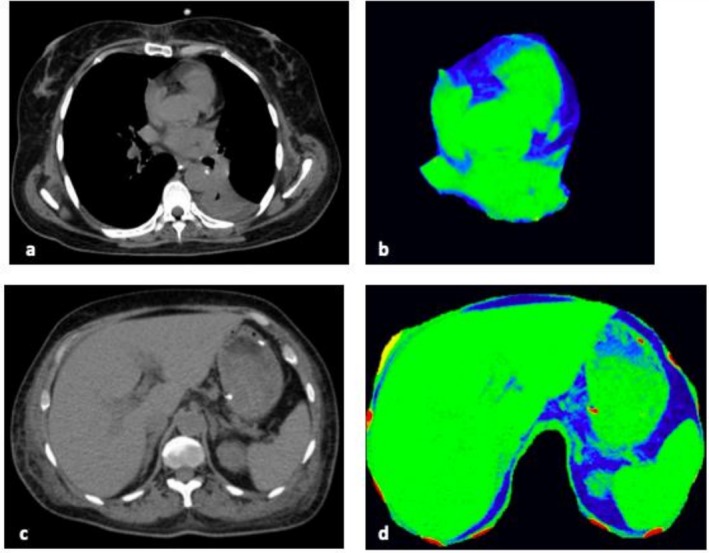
Images from Computed Tomography. Measurement site for epicardial fat at the level of the left coronary artery trunk (a). After adjustment according to Hounsfield Unit density, with fat highlighted in blue (b). Measurement site for visceral fat in the median space of the T12/L1 intervertebral disc (c). After adjustment according to Hounsfield Unit density, with fat highlighted in blue (d).
